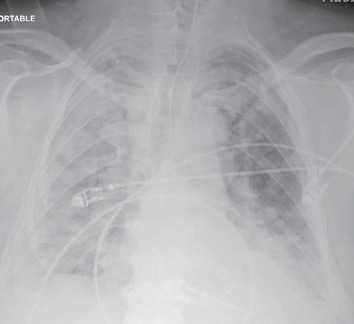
A significant gross increase in diffuse bilateral infiltrates with some sparing of the right upper lobe, which had worsened when compared to previous chest X-rays.
